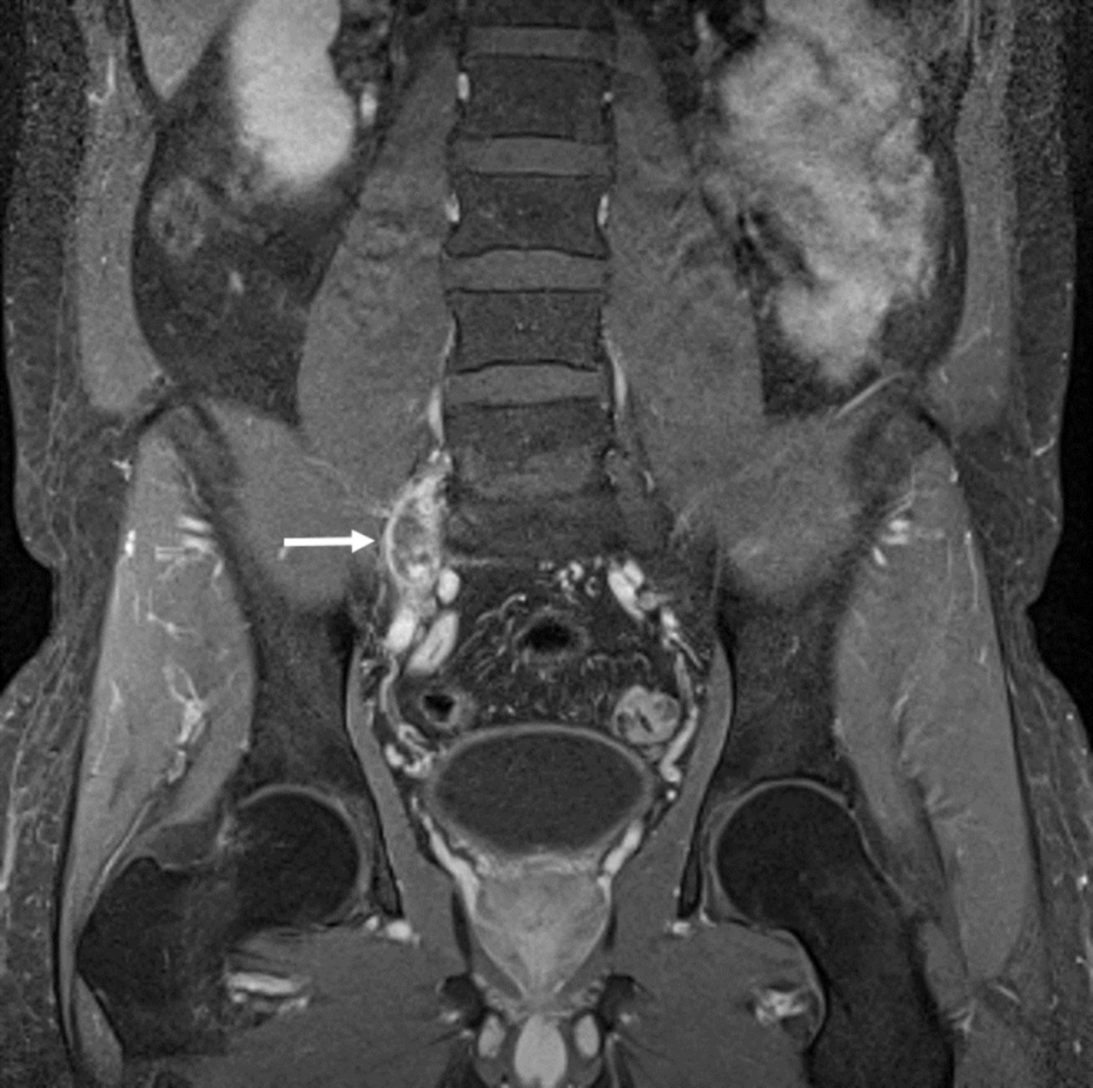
Right pre-sacral mass Coronal fat suppressed contrast-enhanced T1 weighted magnetic resonance imaging (MRI) demonstrating peripheral contrast enhancement of the right pre-sacral mass (white arrow)
